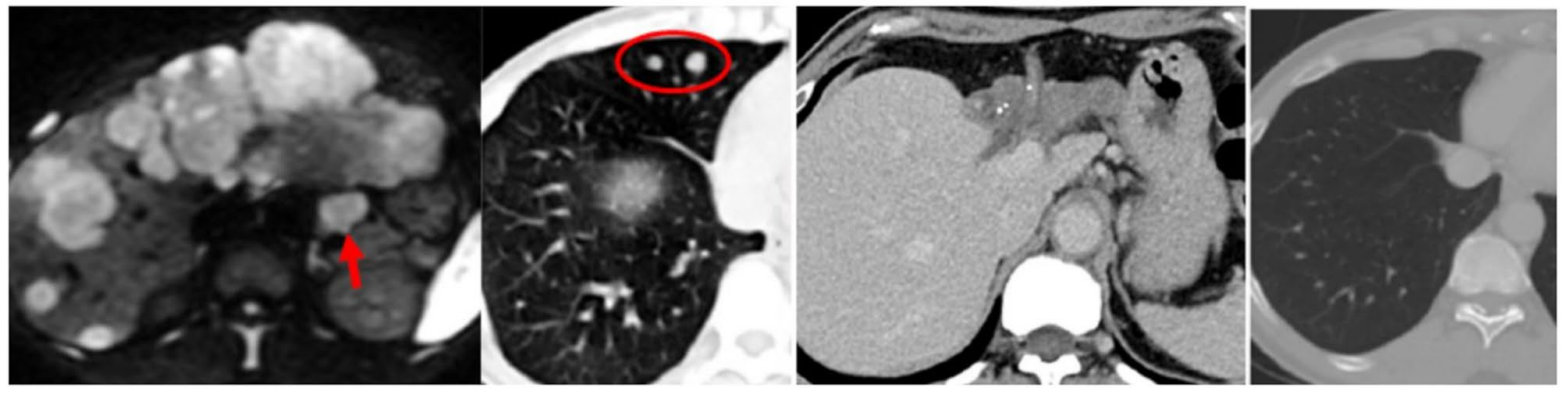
A 50 year-old male advanced HCC patient with extrahepatic spread. A: MRI of the liver (diffusion-weighted image) showed diffuse multiple HCC in the bilateral lobe of liver with a metastatic lymph node (red arrow) in the gastrohepatic ligament. B: CT image of the chest showed several metastatic nodules in the right lung field (red circle). C: CT image of the liver showed complete response of the left lobe lesions with non-visible metastatic lymph node after HAIC neoadjuvant therapy. D: CT image of the chest showed disappearance of the right lung nodules after HAIC treatment. This patient underwent left hepatectomy and resection of the residual viable lesion in the right lobe liver with disease free survival for more than 70 months till the end of the study
